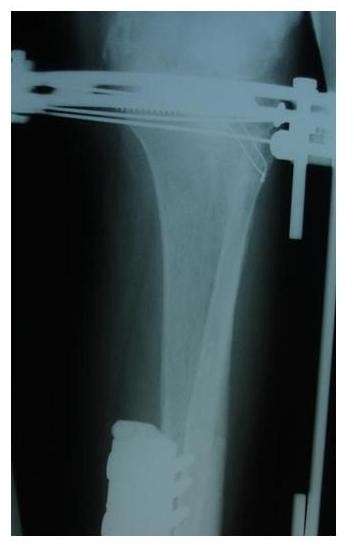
Postoperative AP x-ray of a male patient demonstrating a Schatzker's VI tibial plateau fracture of the left lower limb.
